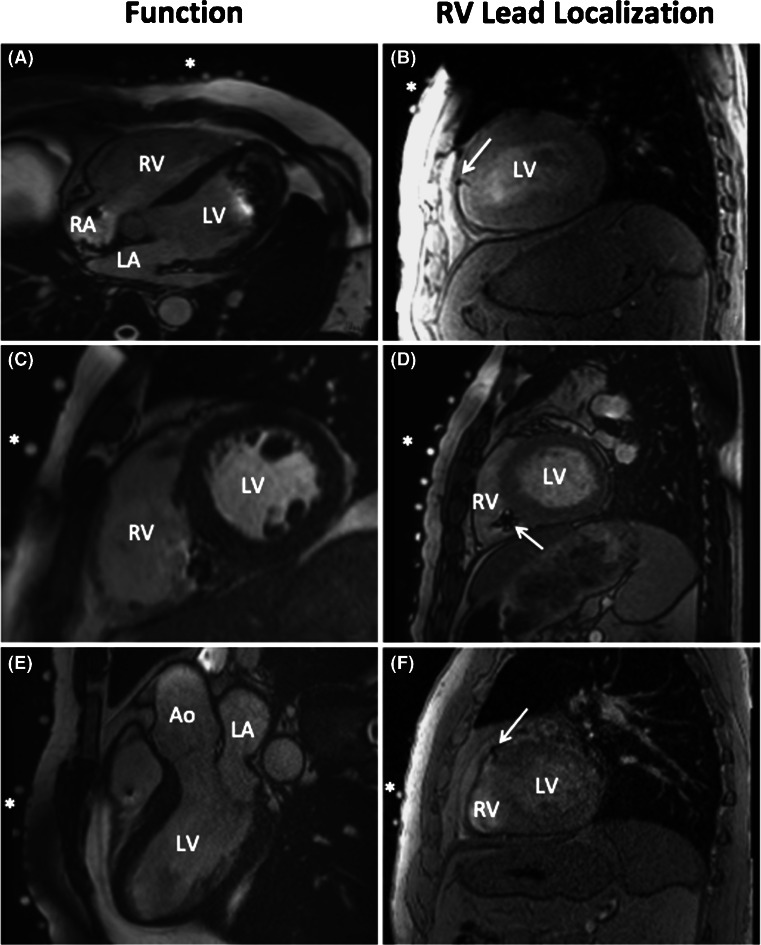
Left panel*, end-diastolic frames from four-chamber (*A*), short-axis (*C*) and three-chamber (*E*) steady state free precession (SSFP) CINE images. Despite regional artefacts, caused by either the impulse generator or the pacing leads, the image quality allows for accurate assessment of LV and RV volumes and function. *LA* left atrium, *LV* left ventricle, *RA* right atrium, *RV* right ventricle, *Ao* aorta. *Right panel*, three examples (*B*, *D* and *F*) of the T2-weighted, bright blood images used to localize the RV pacing lead (*white arrows*). The *asterisks* indicate the MRI markers that represent the location of the BSP electrodes
